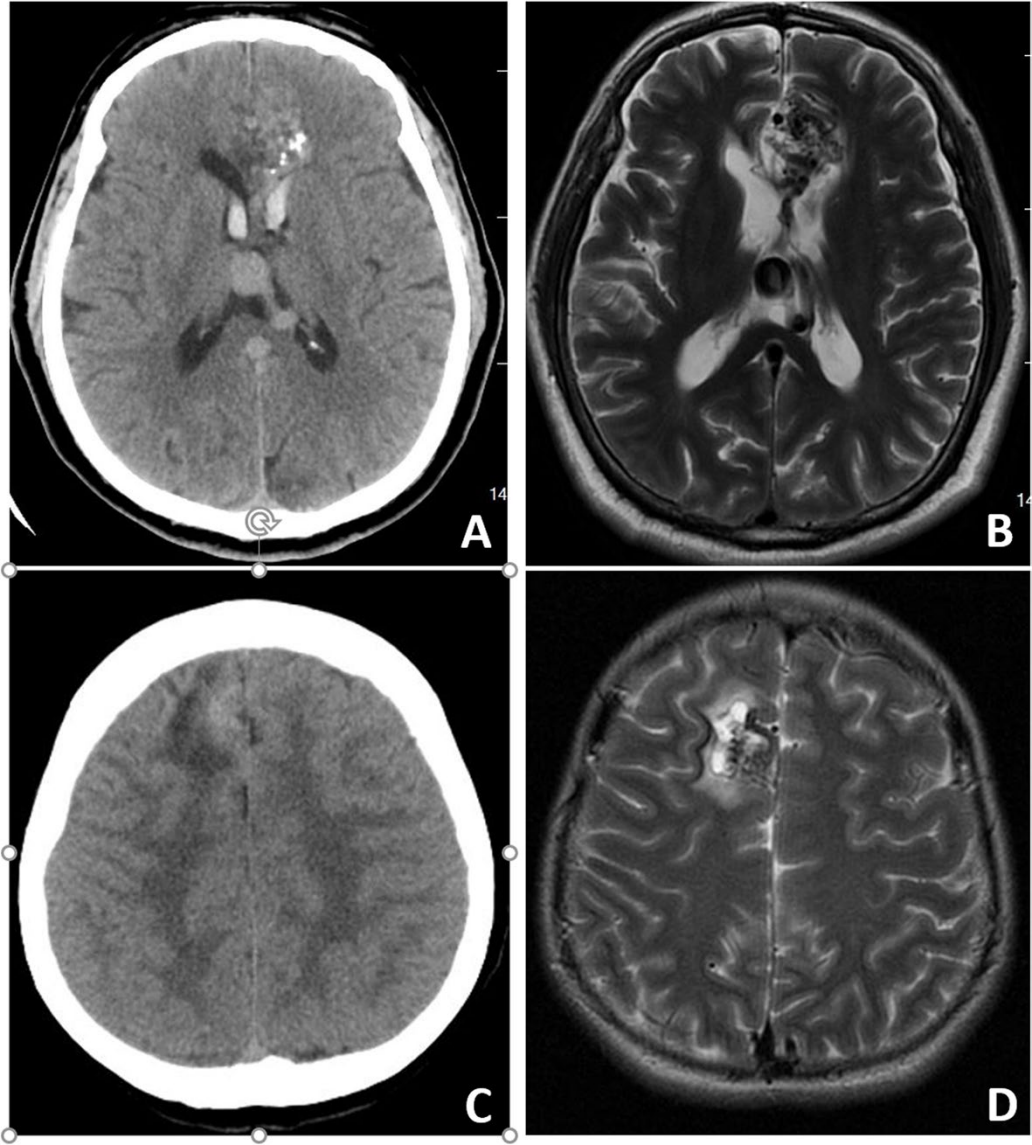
The CT (Additional file 7: Fig. S1A) and MRI (Additional file 7: Fig. S1B) of ruptured BAVM and CT (Additional file 7: Fig. S1C) and MRI (Additional file 7: Fig. S1D) of un-ruptured BAVM
